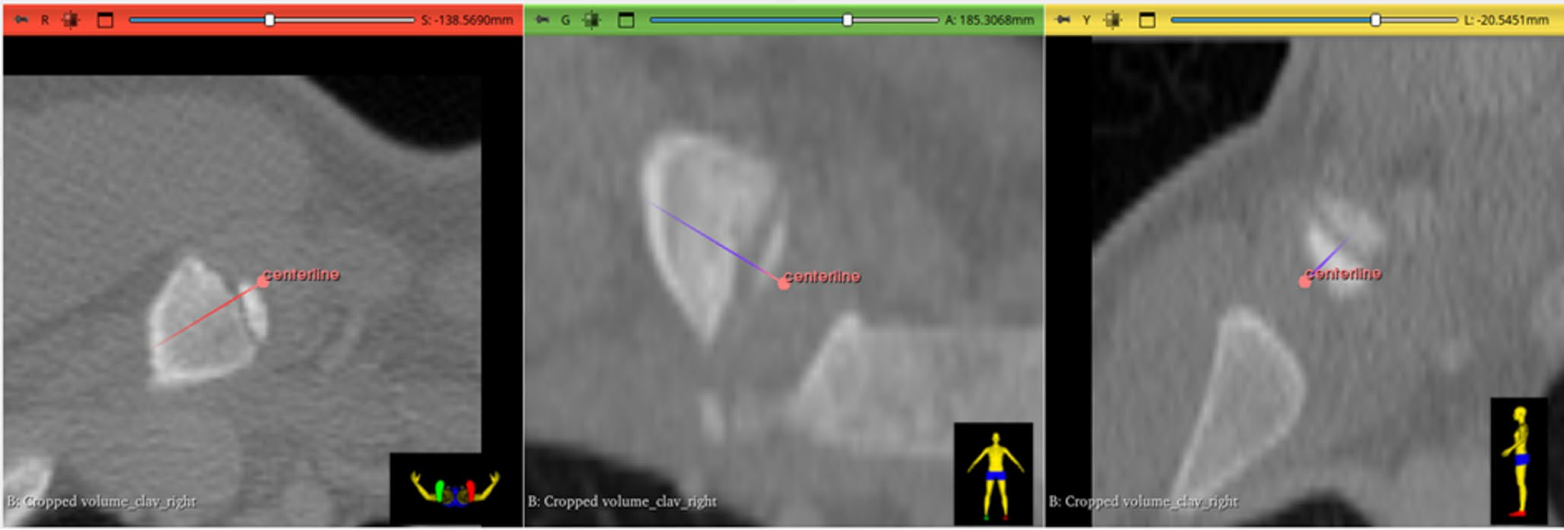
For precise alignment of the 3D model, to obtain an optimal en-face view of the proximal end of the medial clavicle, a centerline was drawn in the CT image. The central position of the proximal endpoint of the centerline anterior to the epiphysis was verified in all three CT image planes. (red: axial view, green: coronal view, yellow: sagittal view)
